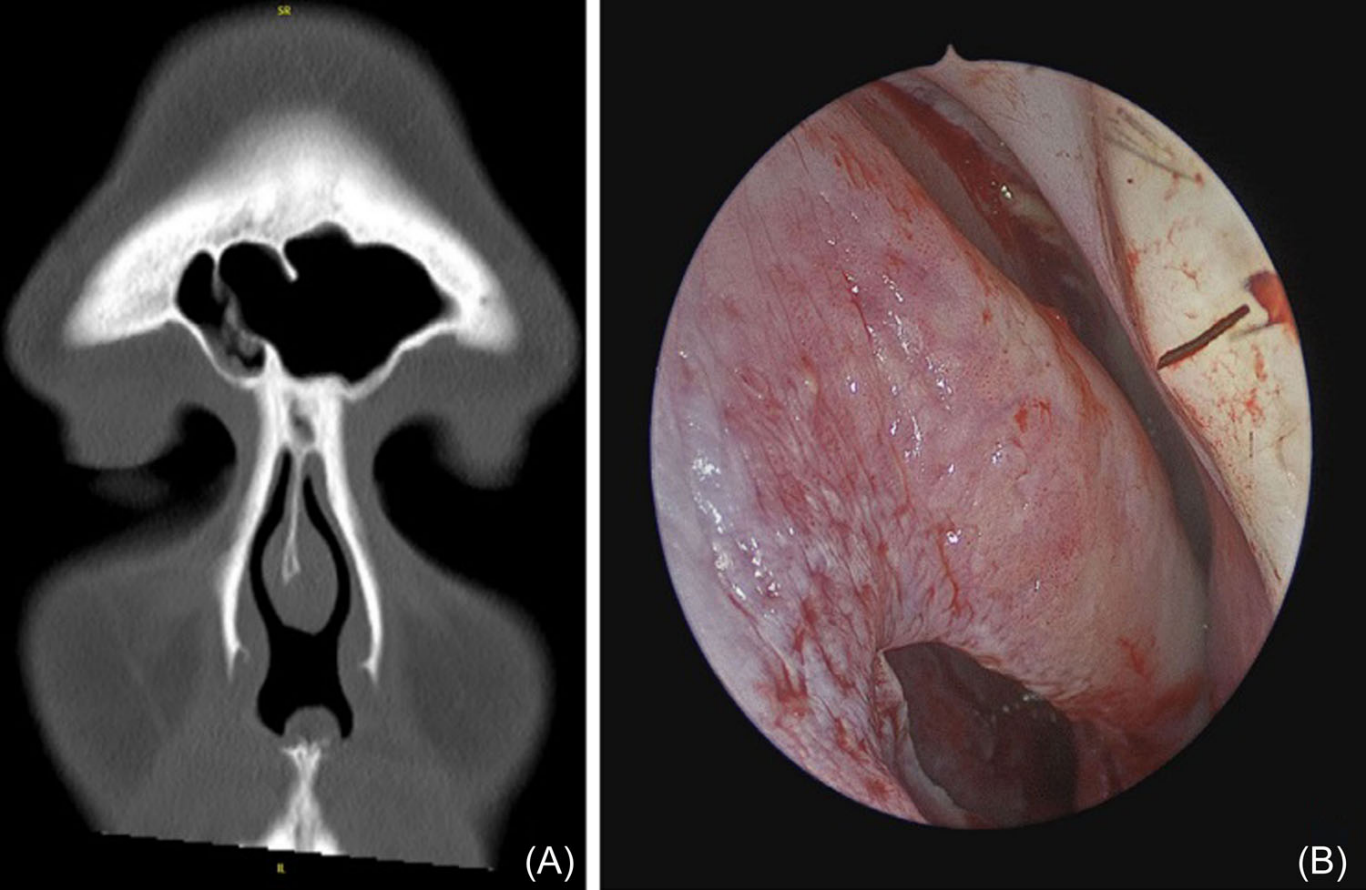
(A) Sinus computed tomography scan demonstrating a thick NSB opposite a high right‐sided deviation superior to a perforation. (B) Intraoperative endoscopic photo of the same patient demonstrating the superior mucosal flap containing the NSB. NSB, nasal swell body.
